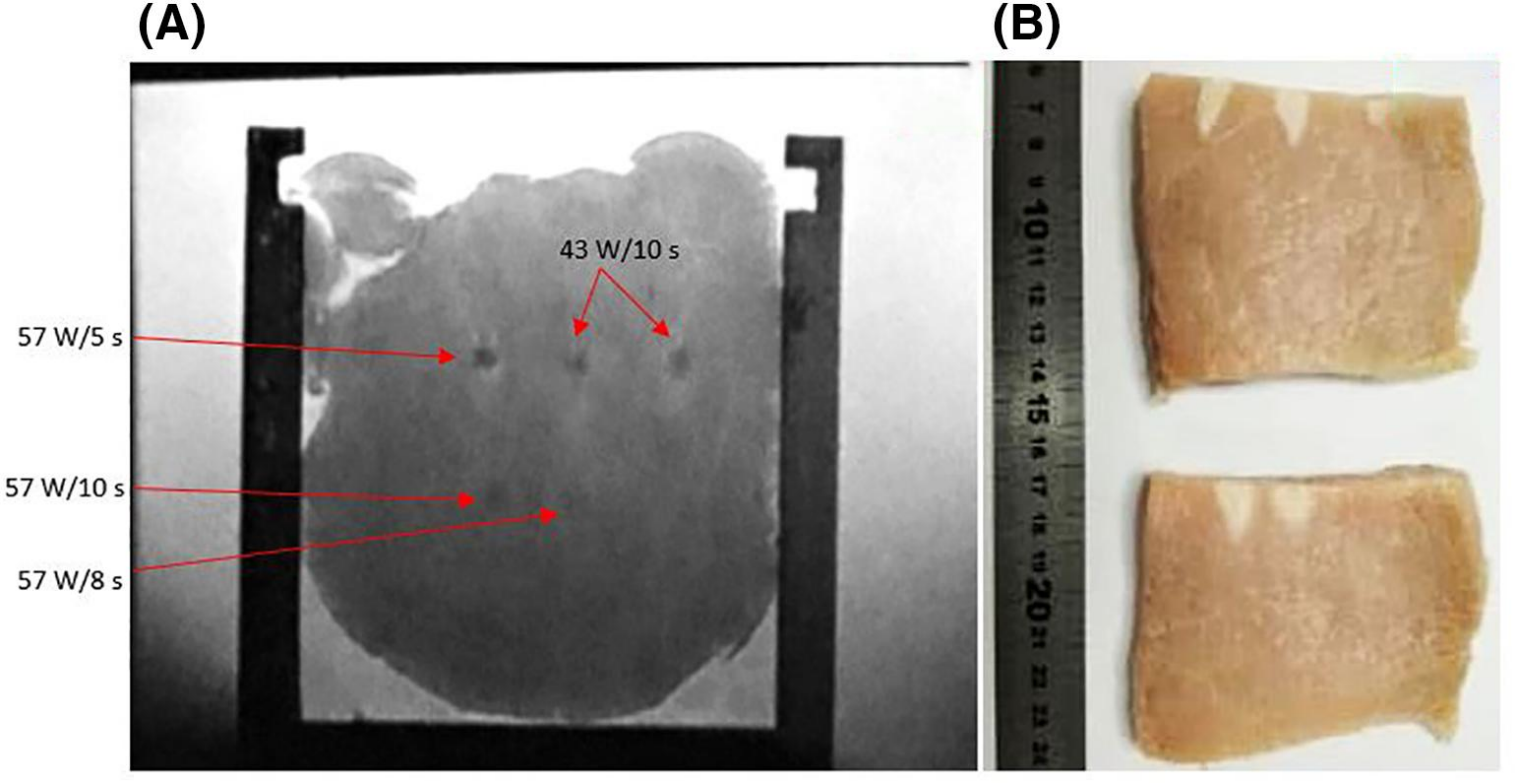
(A) High‐resolution PD with fat suppression MR image (coronal plane) obtained at plane perpendicular to the beam. (B) Sliced tissue with lesions formed at a plane parallel to the beam after sonications with different parameters with transducer having frequency 3.2 MHz at various focal depths. MR, magnetic resonance; PD, proton density
